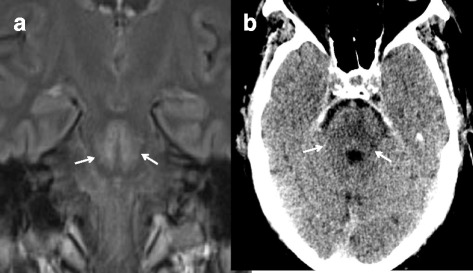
MRI image of the brain (a FLAIR cor) and cranial computed tomography image of the brain (b CE-cranial); arrows indicate the central pontine-demyelinization area
